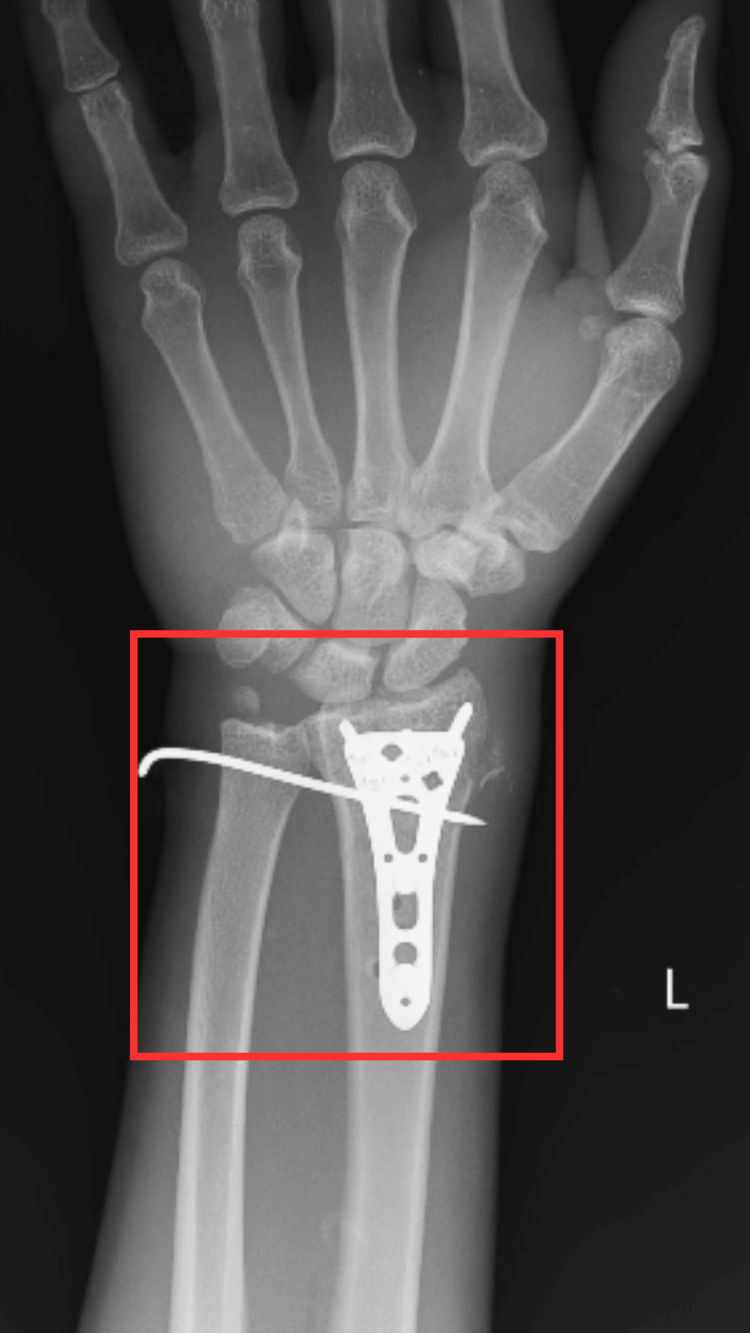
X-ray of the wrist joint The square shows ORIF with plate osteosynthesis for a volar Barton fracture. ORIF, open reduction and internal fixation
